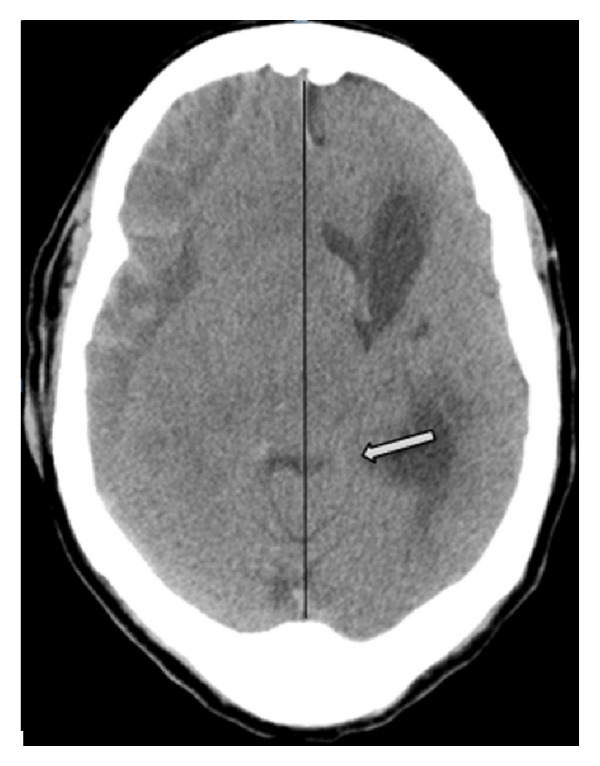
CT of the head showing gross posterior and lateral displacement of midbrain with deformity of the crus cerebri (pointed by the arrow) to the left side. The midline is marked with a black line. The subdural hematoma is visible on the right-side.
